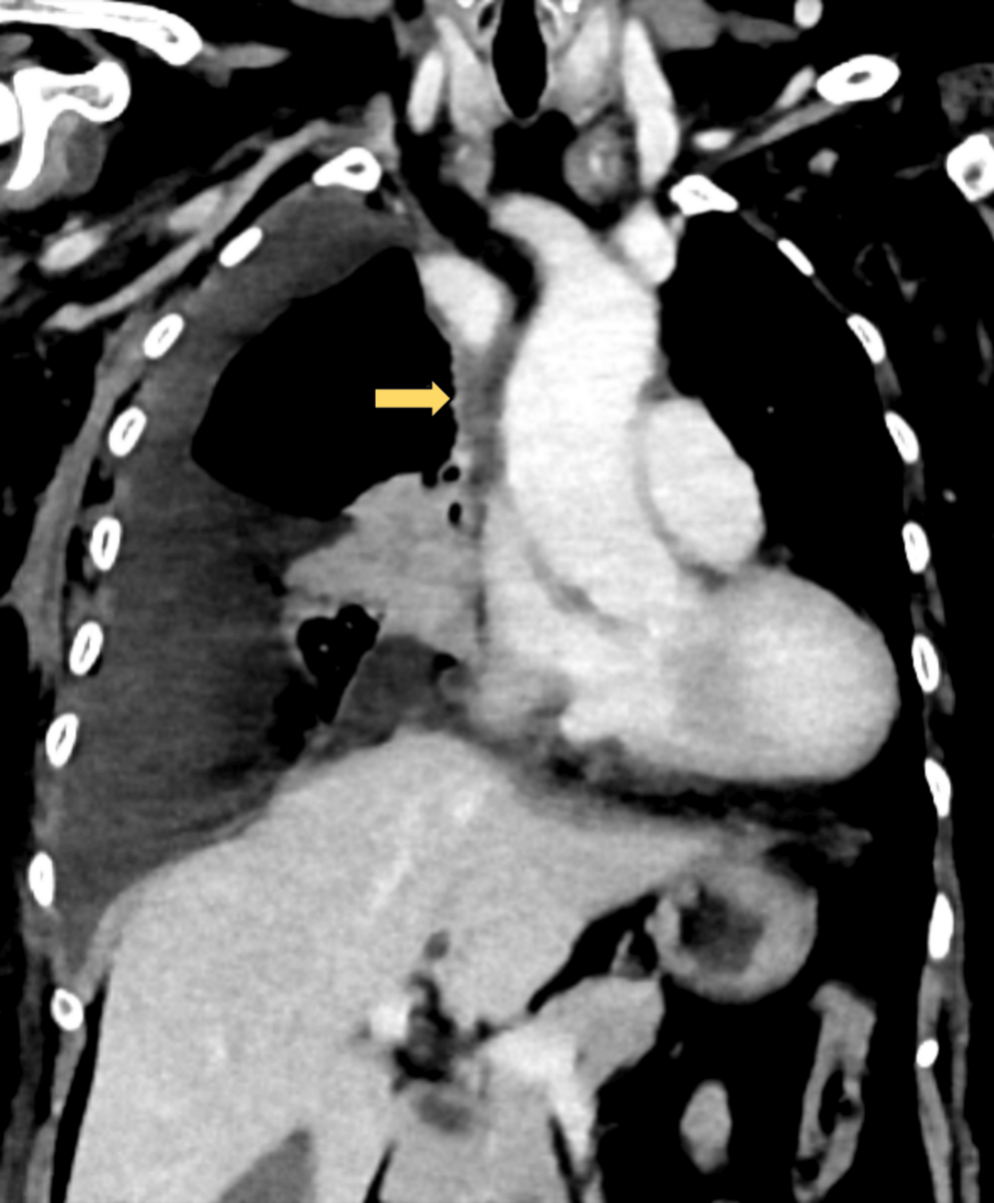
CT venogram demonstrating short segment (~3.3 cm) superior vena cava occlusion with luminal attenuation (arrow) and associated moderate right pleural effusion, subsequently confirmed as chylothorax.
